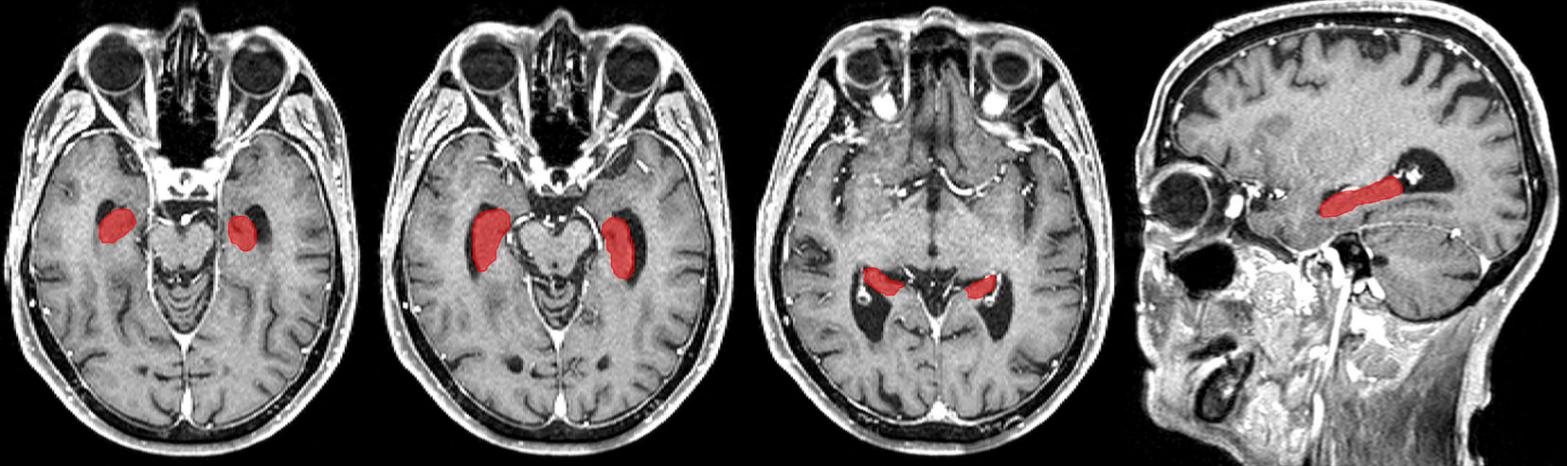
Example of a hippocampus segmentation on CE-T1-MR imaging using the CAT and the *in vivo* high-resolution CoBrA atlas of the hippocampus (26).
